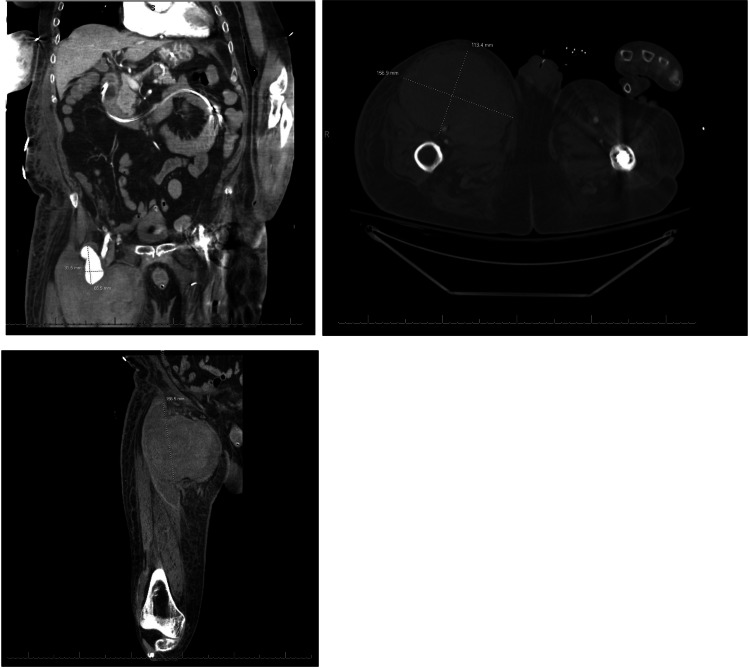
Follow-up CT abdomen/pelvis with contrast Interval increase in the size of the right proximal thigh CFA pseudoaneurysm now measuring 11.2 x 15.4 cm. Small bilateral pleural effusions with left lower lobe consolidation opacity. May represent a combination of atelectasis with the aspiration of infection.
